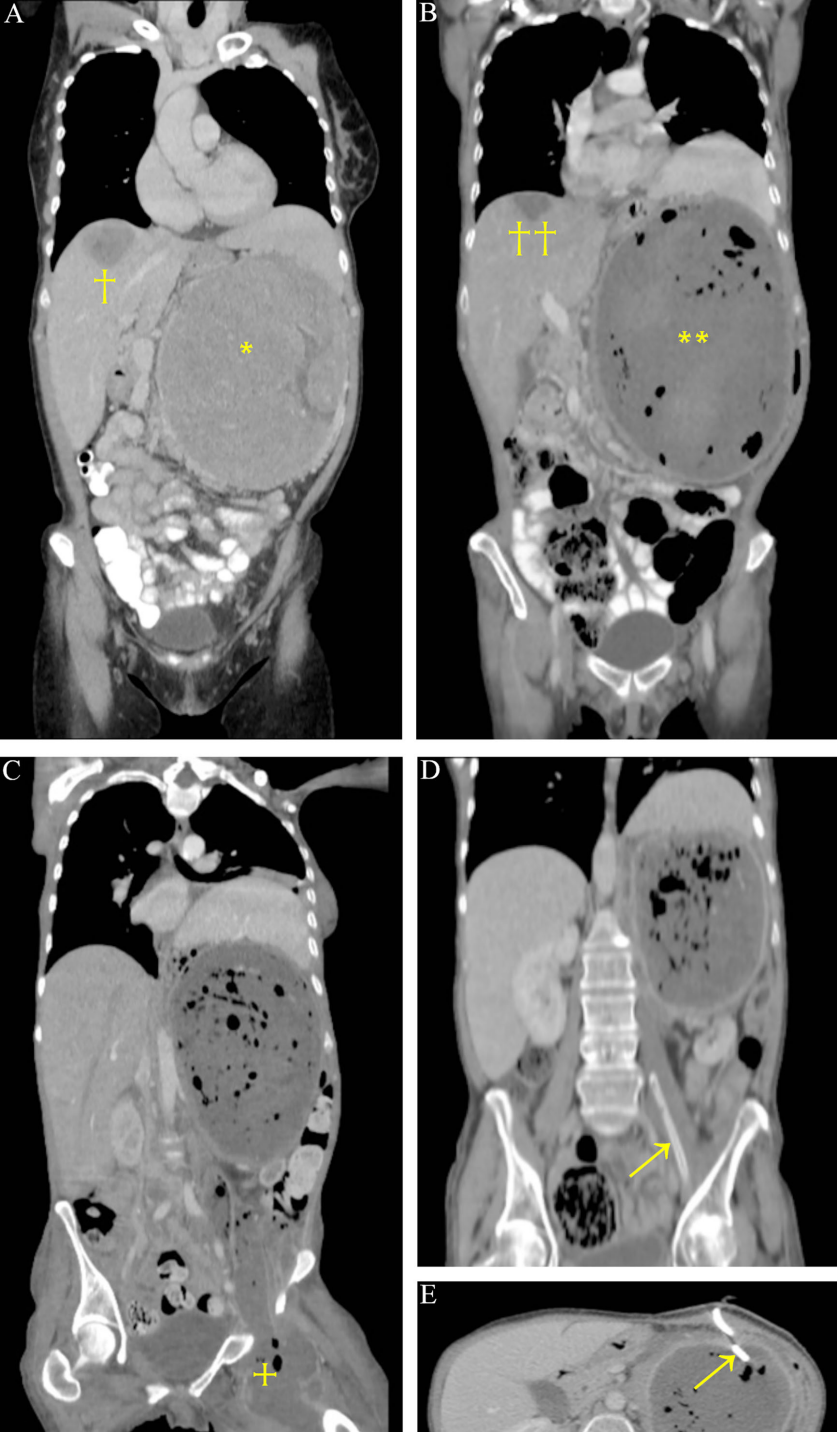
CT scan of 20 cm large left adrenocortical carcinoma (*) with segment 8 liver metastasis (†). The segment 7 tumor is not shown on this reconstruction (A). Necrosis on CT after liver TAE (††) and embolization of the previously bleeding primary tumor () (B). Abscess from the necrotic tumor reaching the thigh (+) (C). Drains in the abscess (arrows); the descending part is already resolved (D, E).
